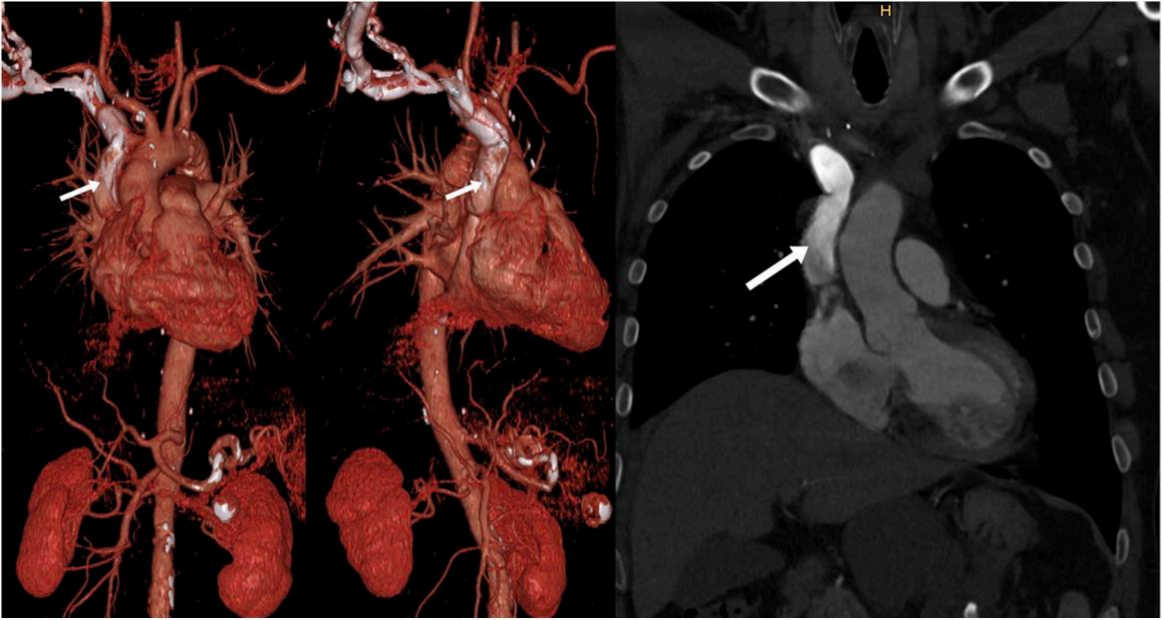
Postoperative CTA at 3-month follow-up demonstrated a satisfactory surgical result with homogenous contrast filling of the PTFE graft without any signs of thrombosis (white arrow).
